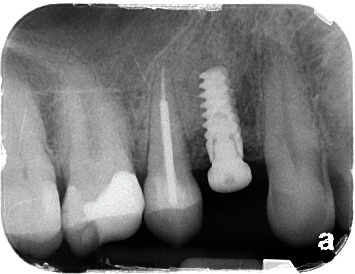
Radiographic healing, showing normal bone density at the 6-month postoperative appointment.
